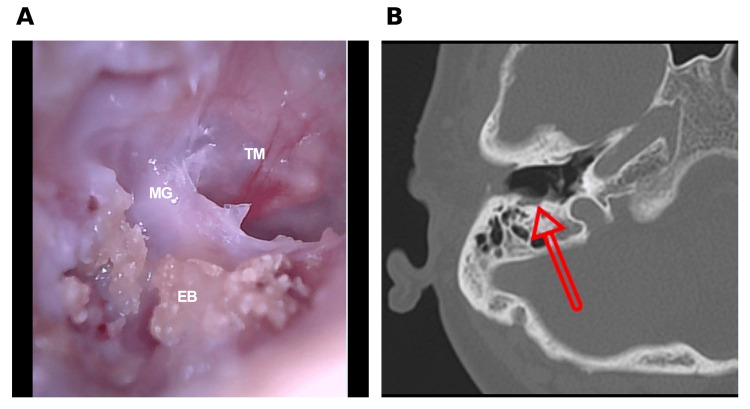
Radiological and endoscopic findings in patient E with benign osteonecrosis of the external auditory canal. (A) Endoscopic clinical image of the EAC showing partial mucosalisation of the inferior indentation and bone exposure. (B) Axial CT of the temporal bone at the level of the inferior EAC, demonstrating indentation of the inferior EAC wall (arrow). TM: tympanic membrane; MG: membrane growth; EB: exposed bone; EAC: external auditory canal.
